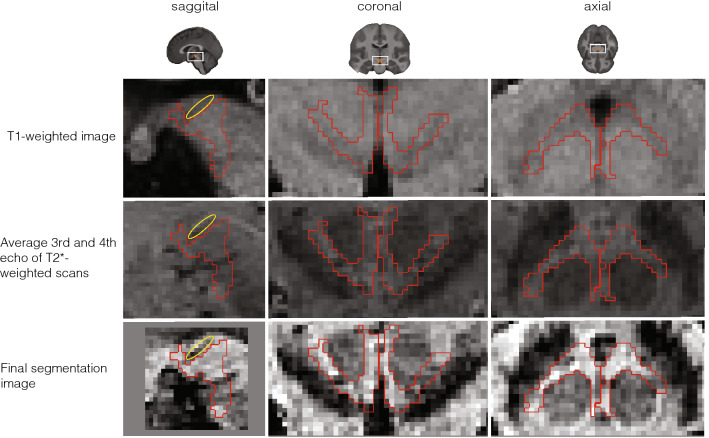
Detail of the midbrain area of one individual participant for each of the different scans collected for VTA delineation. The figure displays VTA (red) for one participant in the sagittal (left column), coronal (middle column), and axial (right column) plane. The first row shows the T1-weighted image of the first inversion of the MP2RAGEME sequence. The second row shows the image based on the average between the third and fourth echo of the T2*-weighted scans. And the third row depicts the final image used for VTA delineation, which was obtained by normalizing the image in the first and second row within the midbrain area (see 2.3). A blood vessel passing through the midbrain is highlighted in yellow, which was avoided during segmentation
